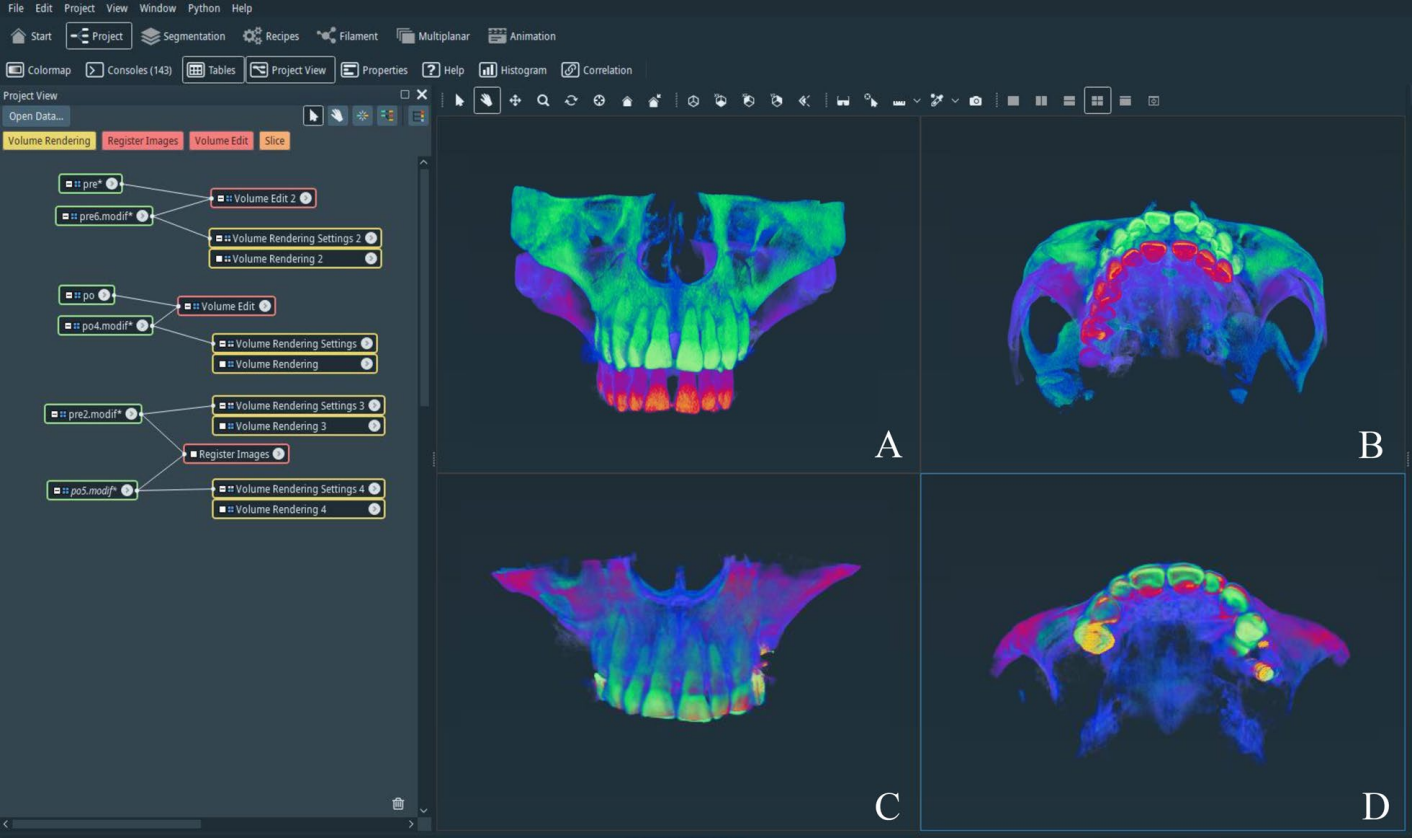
Registration of preoperative and corresponding postoperative CBCT images with 3D reconstruction in the Amira software (Thermo Fisher Scientific Inc., USA). A Coronal view of the preoperative (Red) and postoperative (Green) CBCT images before registration; B Axial view of the preoperative and postoperative CBCT images before registration; C Coronal view of the registered maxilla; D Axial view of the registered maxilla
